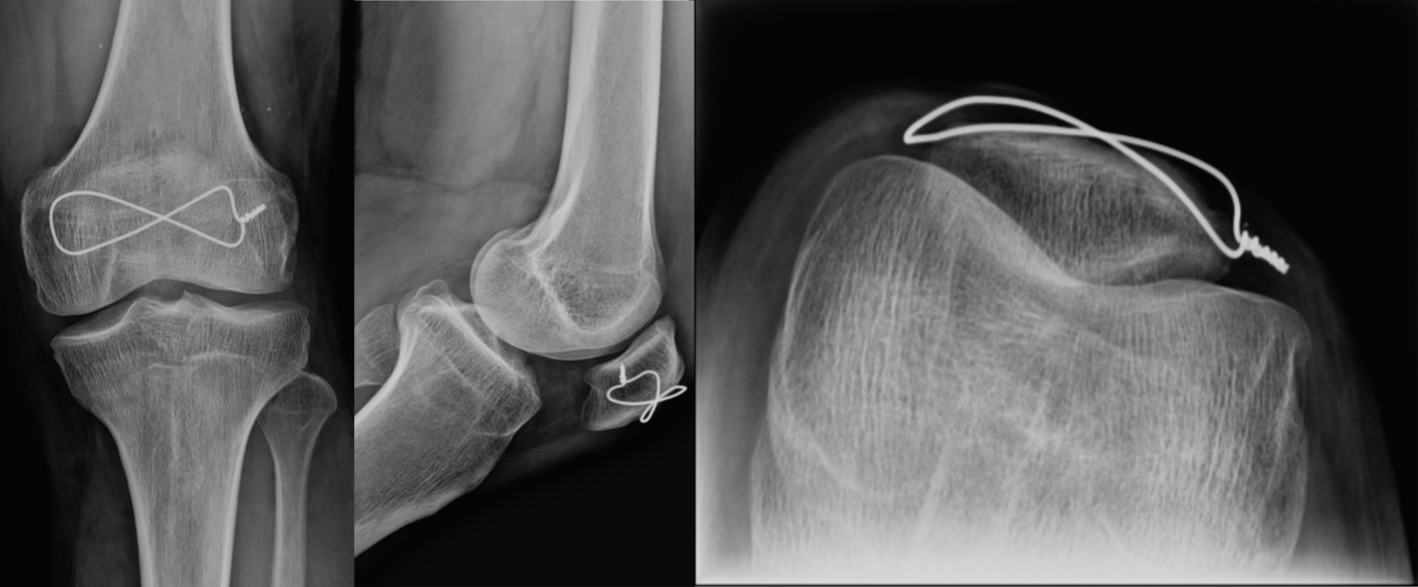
Twelve months postoperatively, no arthritis sign, the complete consolidation without articular gaps and the removal of the K-wires were shown. Anteroposterior radiograph (left side), lateral radiograph (center) and axial radiograph (right side).
